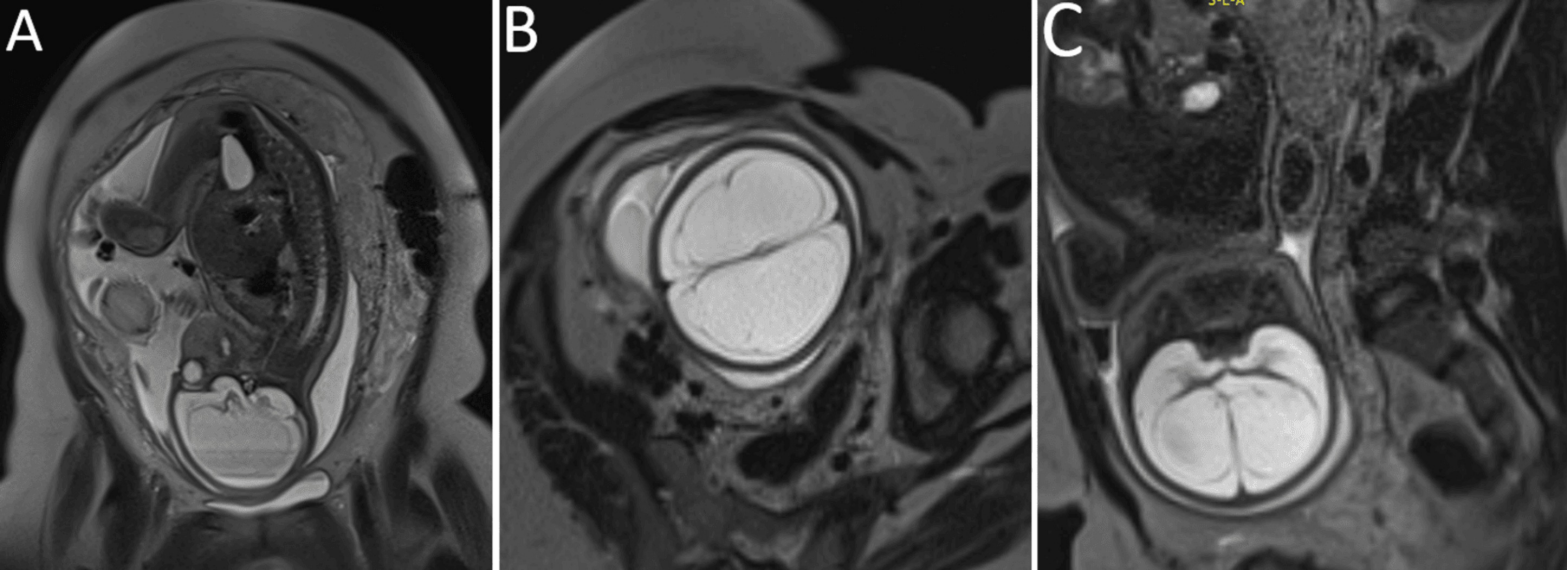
Pelvic MRI, performed in sagittal T2 (A), axial T2 (B), and coronal T2 (C) views, reveals a twin pregnancy The first fetus shows atrophy, indicating a non-viable pregnancy. The living fetus presents with severe bilateral symmetrical hydrocephalus, leading to complete compression and thinning of the cerebral parenchyma.
